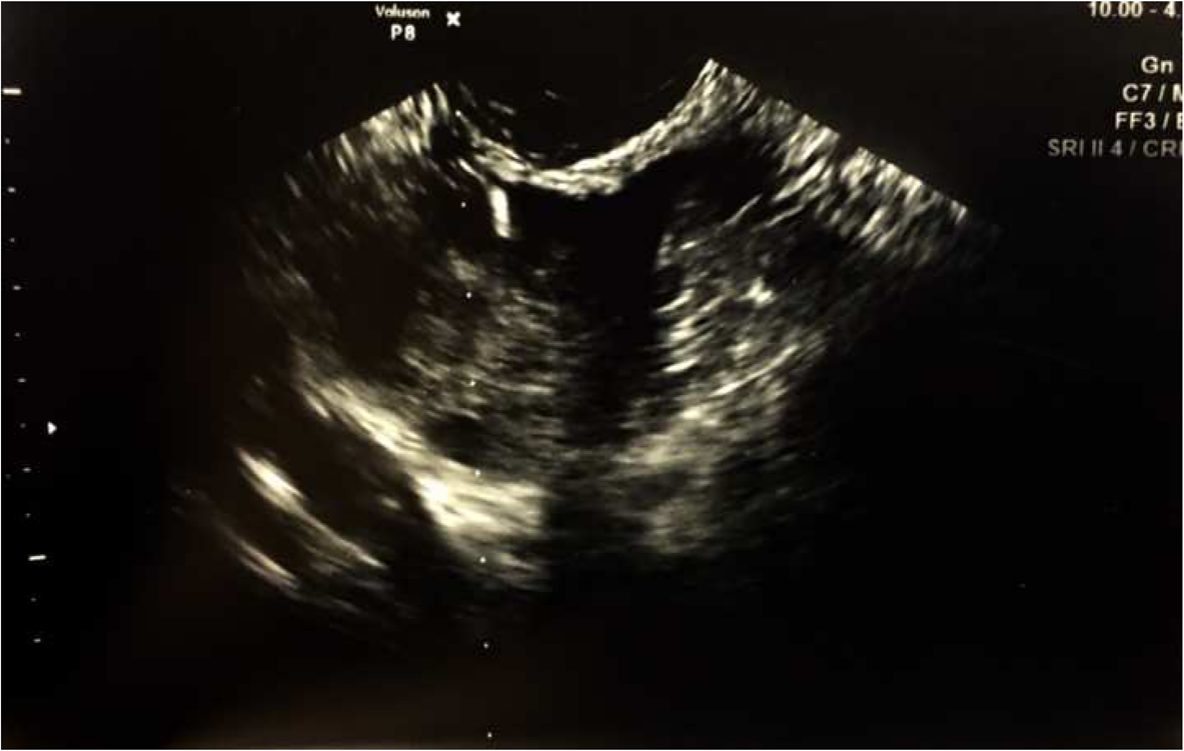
Ultrasound of collapsed follicle after oocyte aspiration
